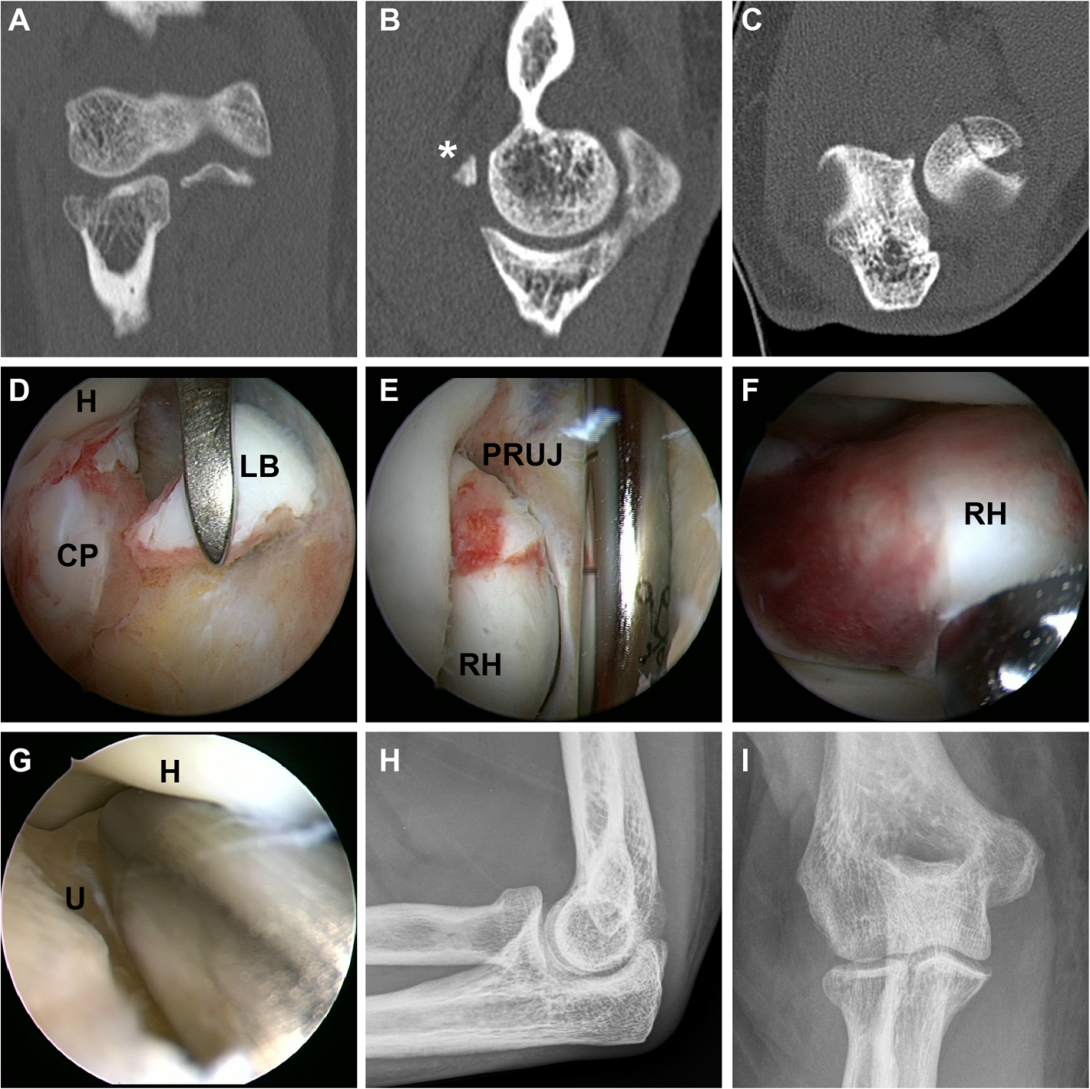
Mason Type I Fracture with loose joint body in the anterior compartment. a-c CT scan showed a mildly (< 2 mm) displaced radial head fracture without blocking of motion and an osteochondral fracture fragment in the anterior elbow compartment. d The loose joint body [LB] was arthroscopically removed after identification between humerus [H] and coronoid process [CP]. e Dynamic evaluation of unimpaired motion in the proximal radioulnar joint [PRUJ] was arthroscopically confirmed. f Exploration of the radial head in full supination. g Posterolateral rotational instability was ruled out with a modified “drive through test” with a switching stick from the soft spot portal between ulnar [U] and humerus [H]. h, i Postoperative x-rays demonstrated correct alignment of the elbow joint and complete removal of loose bodies
